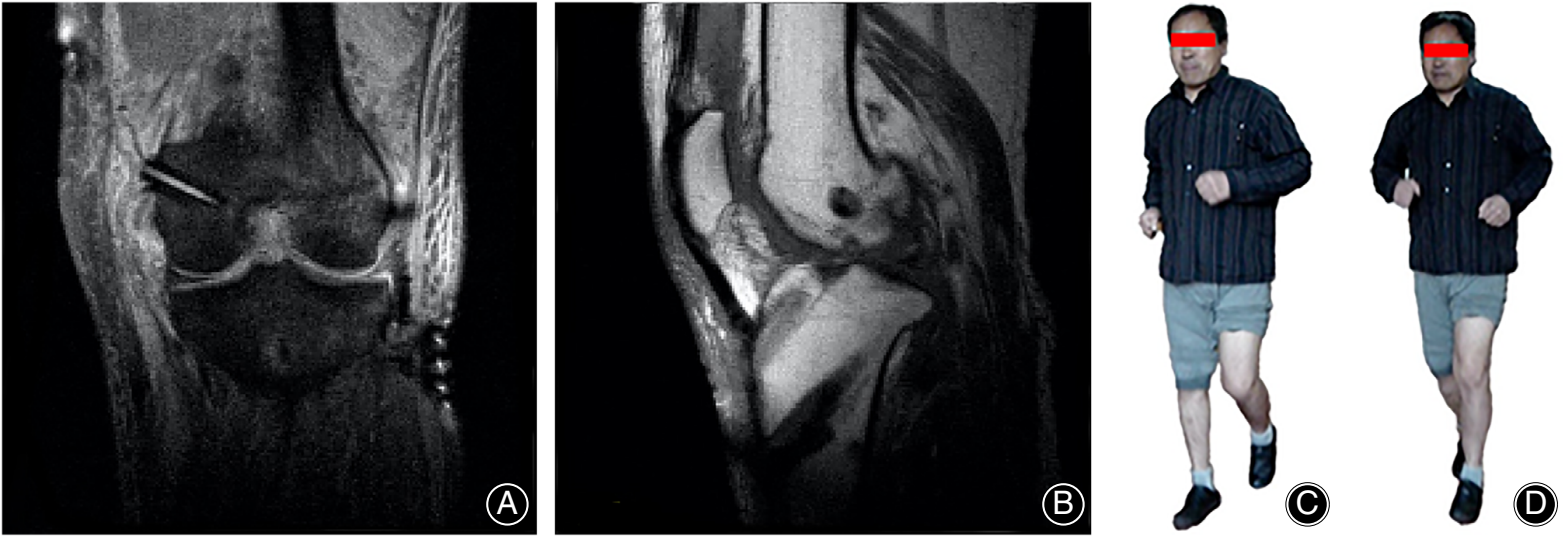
Short‐term follow‐up data. (A, B) Anteroposterior and lateral MRI views of the knee at the 3rd month postoperatively. (C, D) The patient could participate in moderate‐intensity sports 4 months postoperatively
